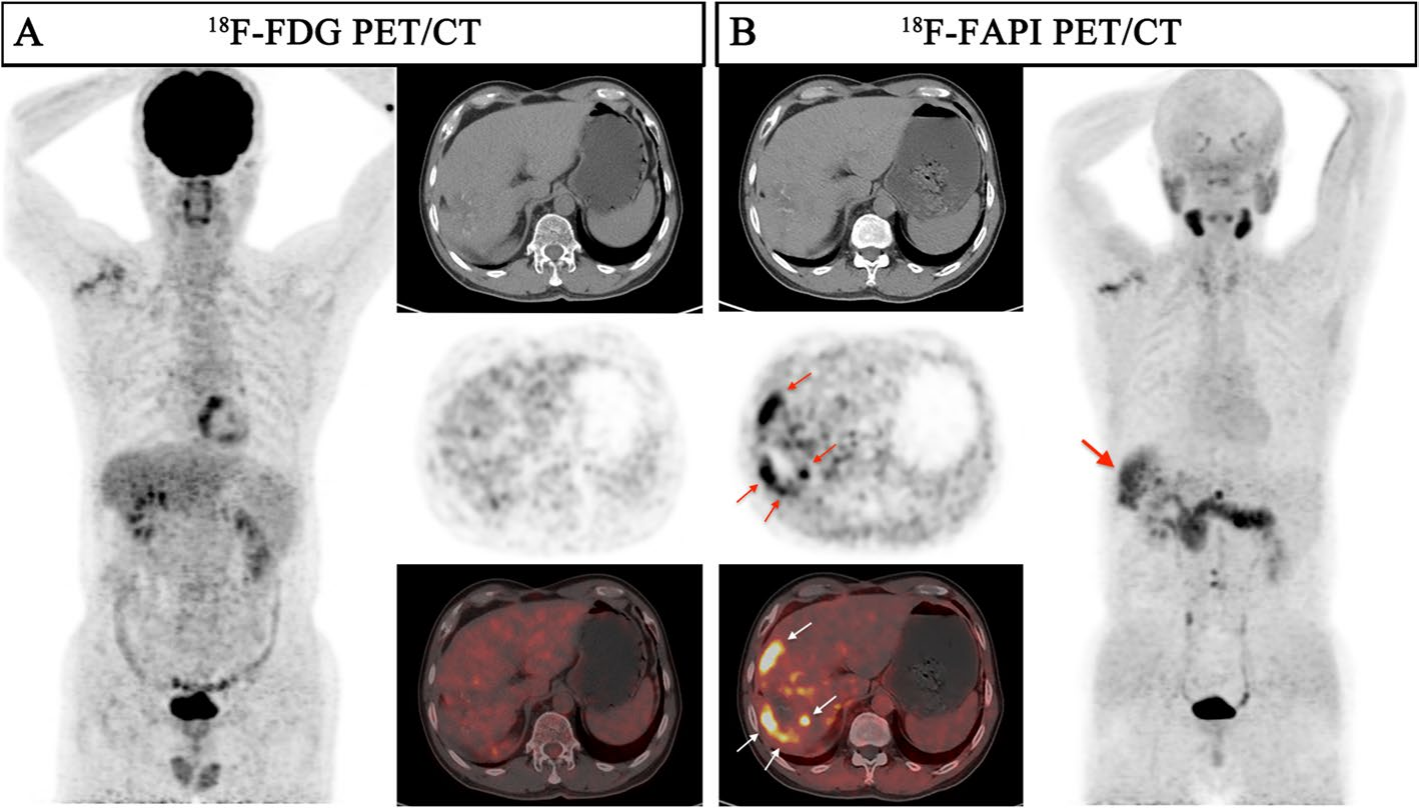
A 57-year-old male patient (Patient No. 50) with recurrent HCC (moderately differentiated) was confirmed by postoperative pathology. ^18^F-FDG PET/CT displayed no uptake in this lesion, although the corresponding CT scan showed lamellar low-density shadow in right lobe of the liver. ^18^F-FAPI PET/CT revealed intense uptake (SUV_max_ 9.0; TBR 6.0) in the recurrent lesion on both maximum intensity projection (MIP) (large arrow) and axial images (small arrow)
